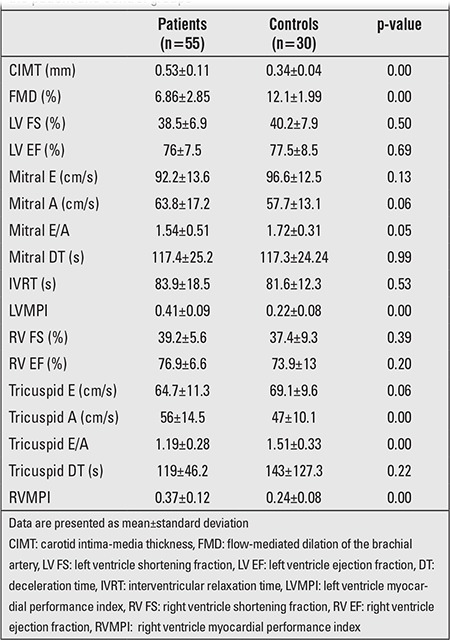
Pulsed-wave Doppler and B- mode echocardiographic data inthe patient and control groups
